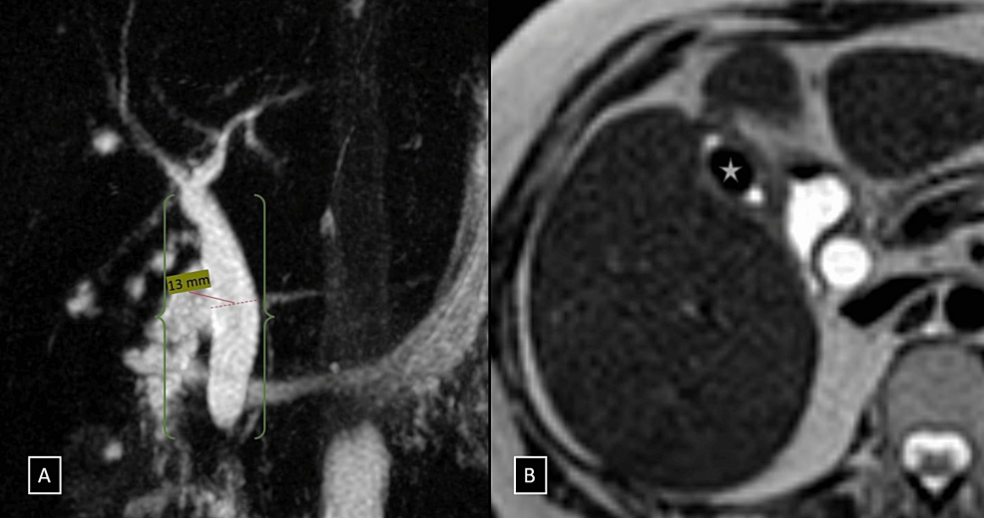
(A and B) Coronal MIP images from heavily T2-weighted pulse sequences showing a dilated CBD with abrupt tapering just proximal to the ampulla of Vater (denoted by a green brace in figure A). Axial sections of T2-weighted imaging showing a large ovoid calculus measuring 13 × 9 mm within the lumen of the gallbladder (white star in B). CBD, common bile duct
